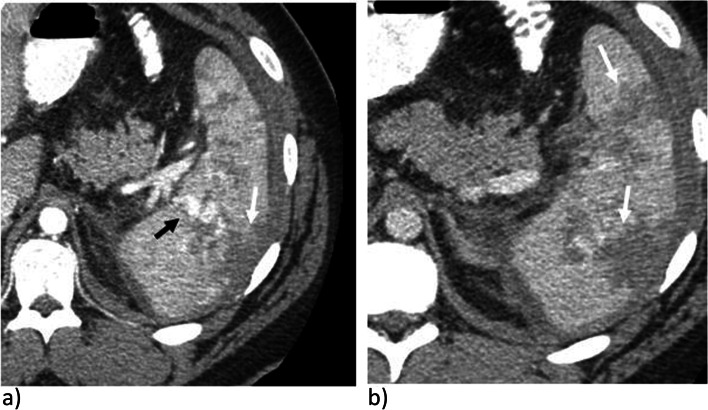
CT in a trauma patient in a) mixed arterial and portal venous phase in a dual bolus injection and b) delayed phases demonstrating a grade IV splenic injury with large lacerations (solid white arrow) and pseudoaneurysms (solid black arrow). There is no extravasation of contrast as confirmed on
